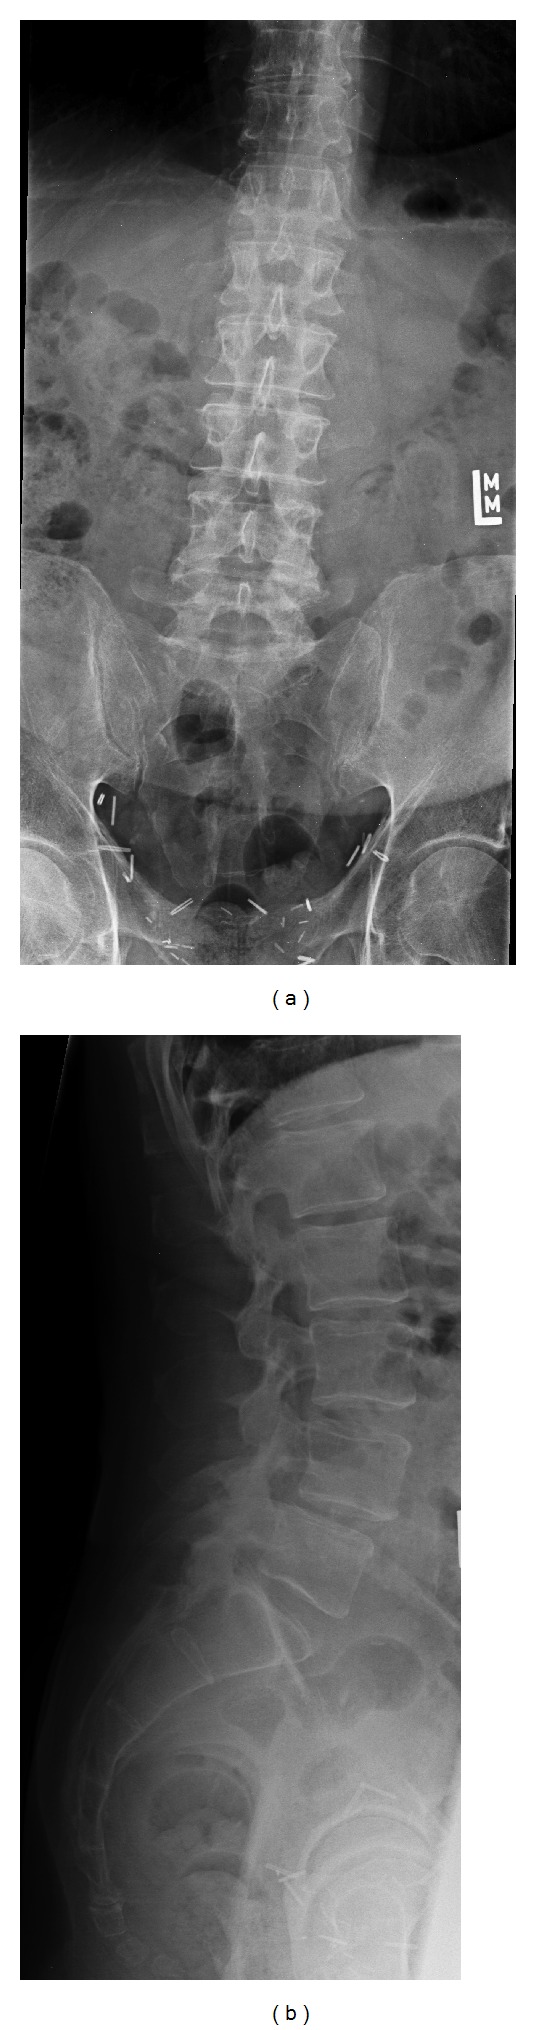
(a) and (b) Anteroposterior and lateral radiographs of a 63-year-old gentleman with severe left lower extremity pain unresponsive to conservative measures. Workup revealed him to have an L4-5 degenerative spondylolisthesis in addition to having a left-sided juxtafacet cyst compressing the descending root of L5. The patient underwent L4-5 Dynesys placement in addition to laminoforaminotomy, mesial facetectomy, and excision of the facet cyst.
